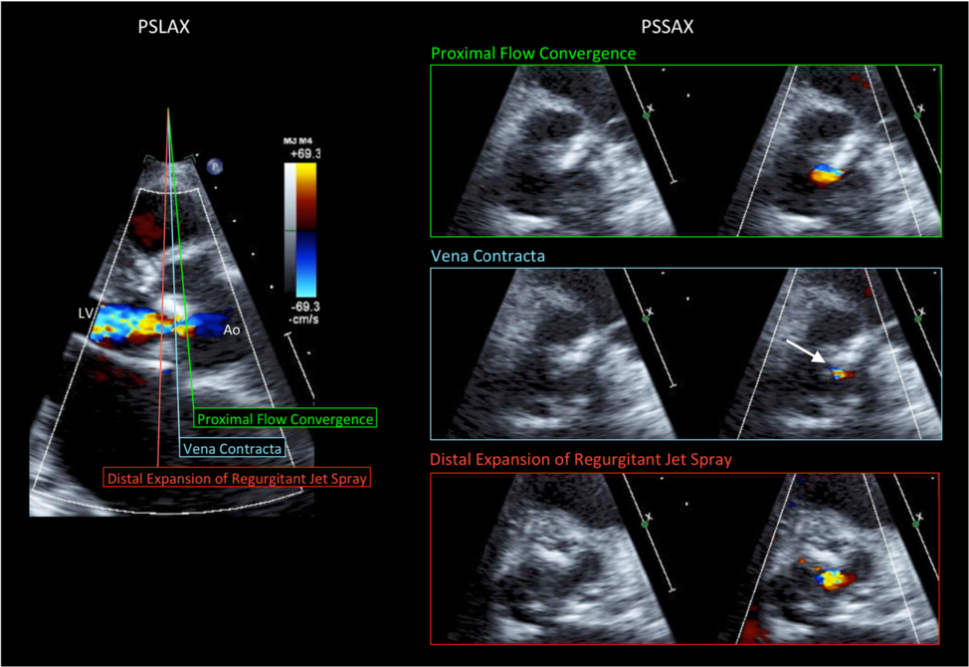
Measurement of two-dimensional vena contracta area (2DVCA) using two-dimensional color Doppler imaging. Parasternal long-axis view (left). Cross-sectional plane of the proximal flow convergence (right, top); Cross-sectional plane of the vena contracta (right, middle). The narrowest area of the jet was measured as 2DVCA (arrow). Care was taken not to include the surrounding blurred color signals as VCA by checking both color and color-suppressed images. Cross-sectional plane of the distal expansion of the regurgitant jet spray (right, bottom). PSLAX, parasternal long-axis view; PSSAX, parasternal short-axis view; Ao, aorta; LV, left ventricle.
